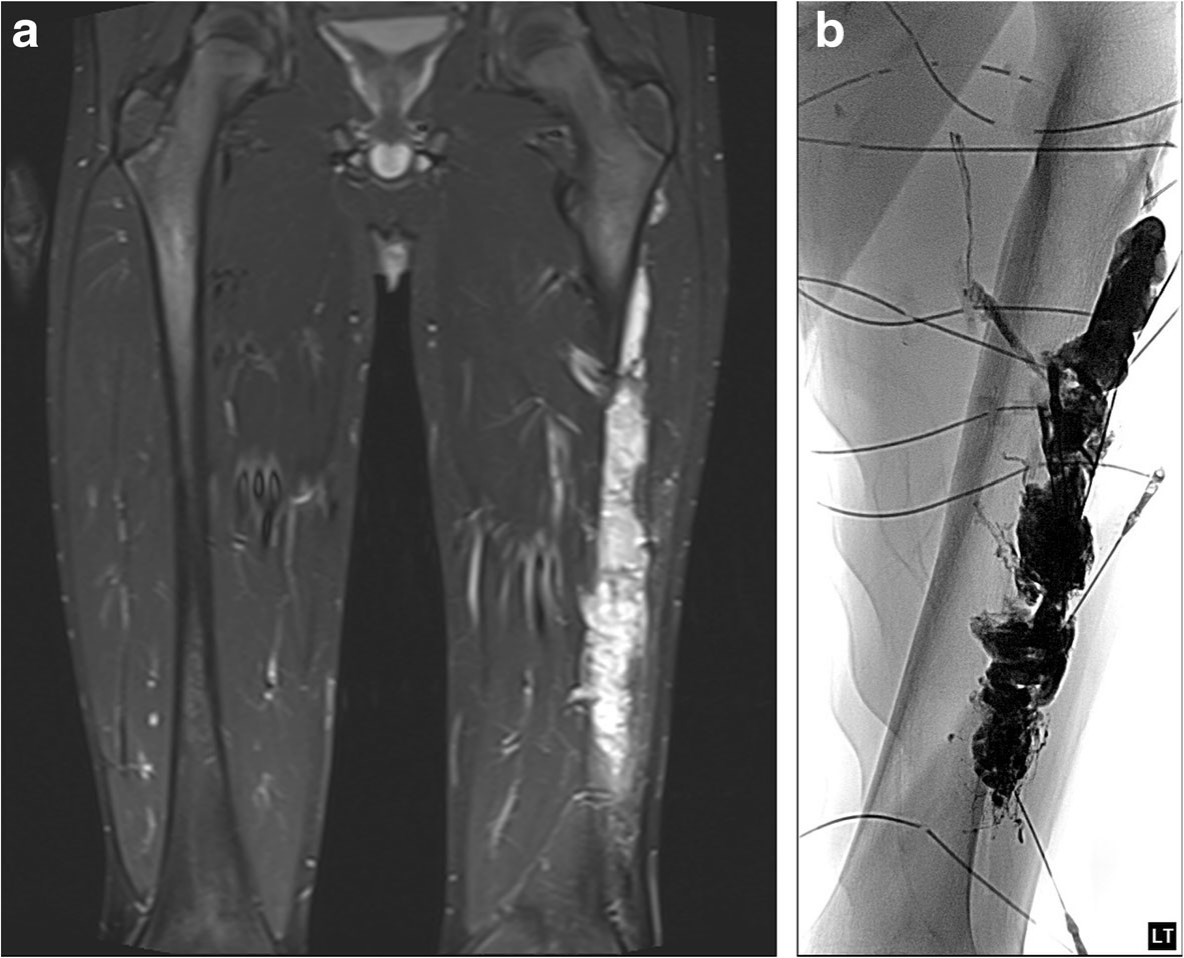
a Pre-operative coronal T2 weighted MRI in a 14-year-old male with a large (2.7 × 3.2 × 21.2 cm) intramuscular left vastus lateralis VM. He had undergone 3 prior stand-alone excisions but had persistent symptoms and large residual VM. b Post-embolization frontal spot image showing *n*-BCA glue embolization of the lesion, using multiple points of percutaneous access
